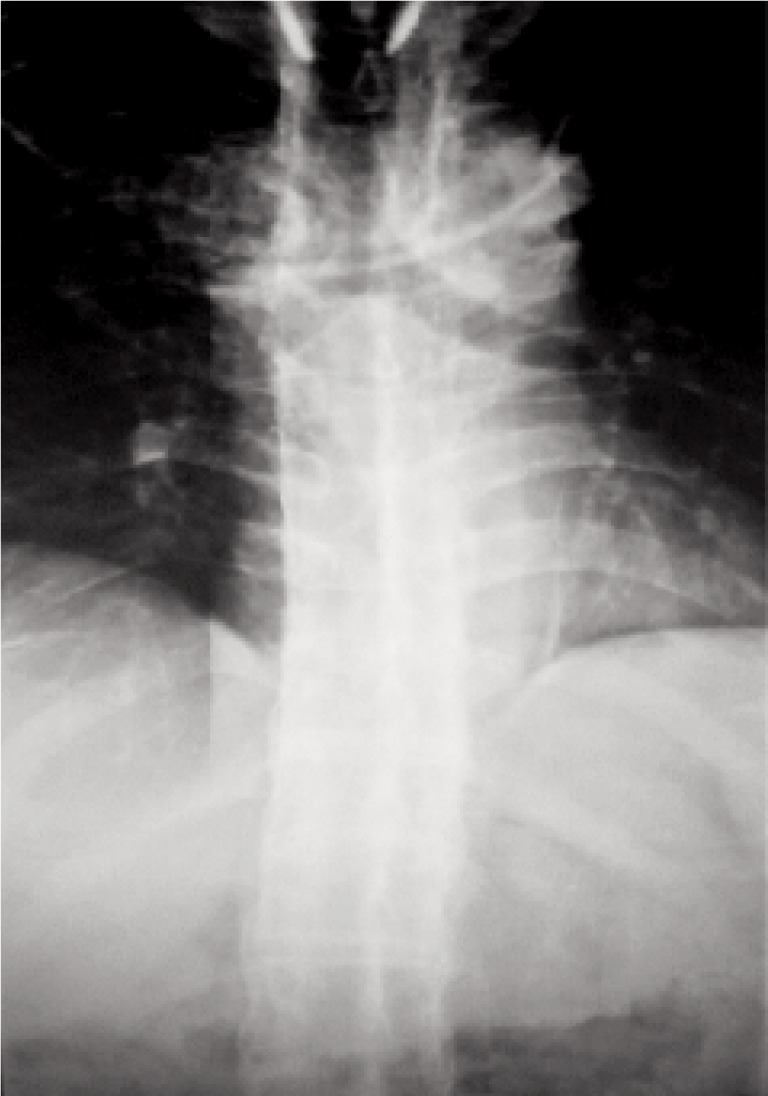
Plain radiograph showing “bamboo spine.”
